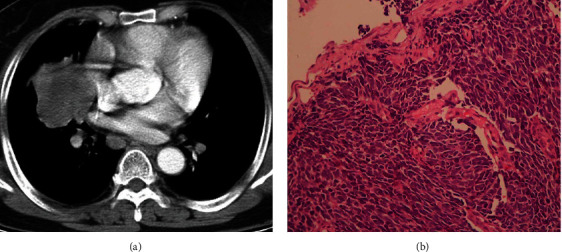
The CT image (a) and pathology (b) from a patient with small-cell lung cancer.
